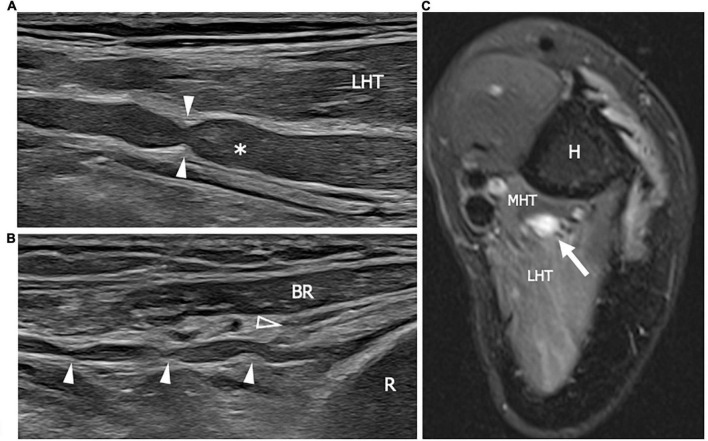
This figure shows two 22 MHz HRUS images (A,B) and one T2-weighted with fat saturation axial magnetic resonance image (C) in a 41 years old patient who developed a Parsonage-Turner syndrome following the vaccination against COVID-19. (A) The radial nerve (white asterisk) is seen in its long axis. The nerve results enlarged with a focal stricture (white arrowheads) along its course, deep to the lateral head of the triceps, proximal to the humerus spiral groove. The stricture is a sign of a torsion neuropathy, typically described in association with Parsonage-Turner syndrome. Multiple narrowing points (white arrowheads) can be observed also along the posterior interosseous nerve (PIN) course in (B). The long axis view of the PIN shows the pathological alterations in the nerve tract proximal to the Frohse arcade or the supinator arch (empty arrowhead) which represents the likely fixation point predisposing to PIN torsion. (C) The radial nerve appears enlarged with an increase T2-signal as a consequence of intraneural edema. Notice the slight T2-signal hyperintensity of the lateral head and medial head of the triceps (LHT and MHT, respectively) related to muscle denervation. H, humerus; R, radius; BR, brachioradialis muscle.
